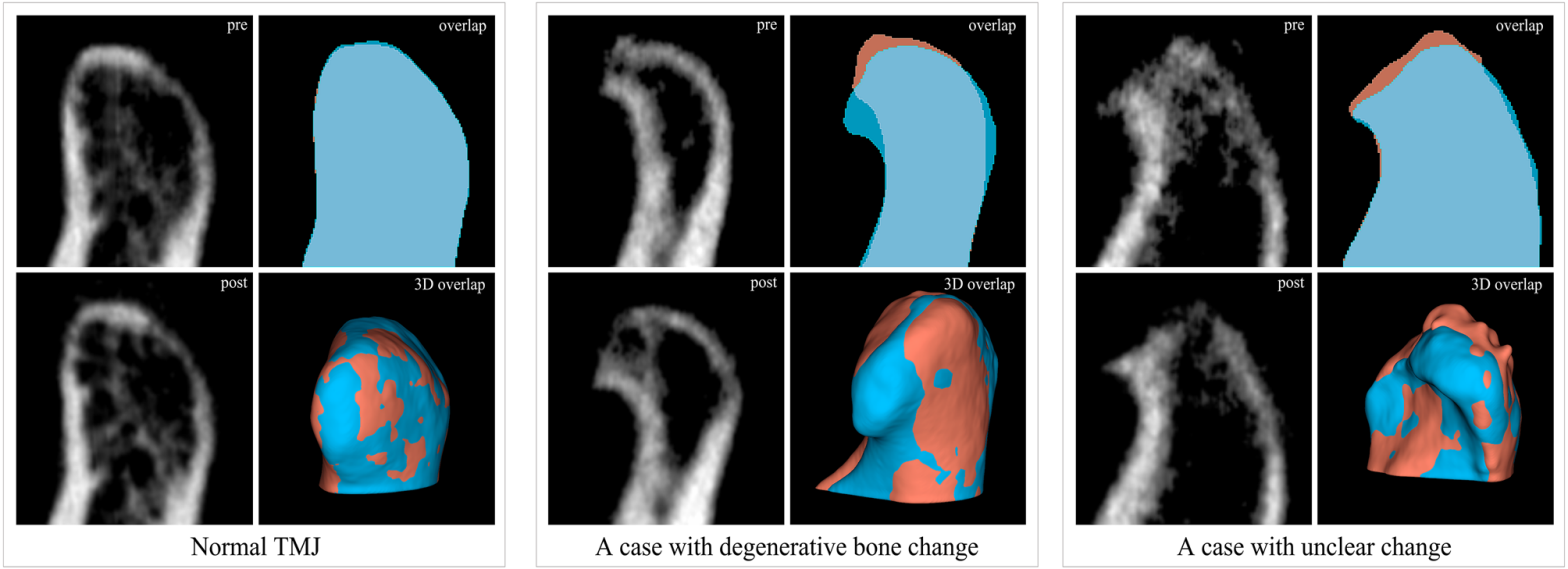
Representative images of temporomandibular joint (TMJ) cone-beam computed tomography (CBCT) images and image processing result. Sagittal CBCT images of pre-treatment (upper row) and post-treatment (lower row) condyle from a normal subject and patients with degenerative joint diseases. Overlap visualizes the comparison of segments between pre- and post-treatment condylar heads. Red segment, pre-treatment; Blue segment, post-treatment. 3D overlap shows rendered models of pre- and post-treatment condyle. Red segment, pre-treatment; Blue segment, post-treatment. The images were generated using the open-source software 3D Slicer (v4.11, Slicer, http://www.slicer.org/).
